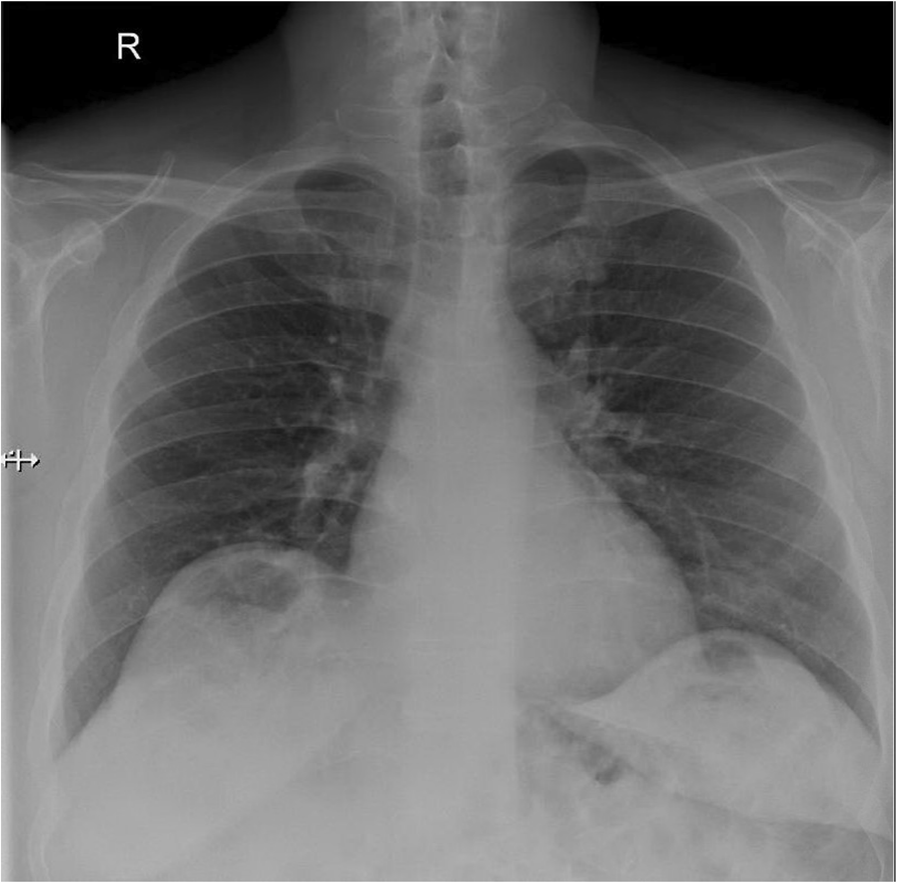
Chest X-ray shows air under the diaphragm
